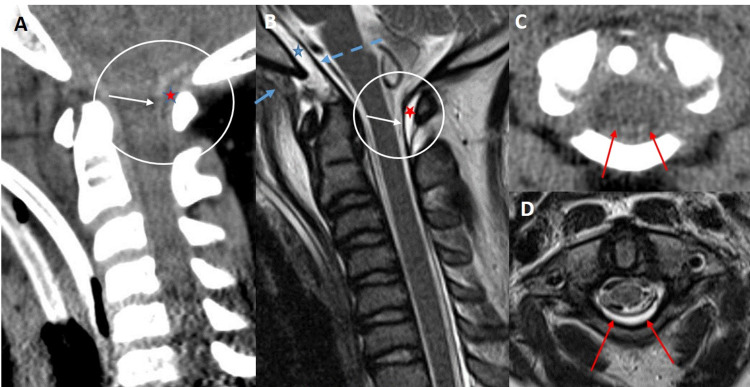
9-year-old female status post motor vehicle accident presenting for cervical CT and MRI exam. Sagittal CT (A) and T2 weighted MRI (B) of the craniocervical junction demonstrate a stripping injury of the posterior atlanto-occipital membrane from the posterior C1 arch (white arrow) with a localized posterior epidural hematoma at C1-C2 (red star). Associated stripping injury of the tectorial membrane (blue dashed arrow) from the posterior clivus with a retroclival epidural hematoma (blue star) with posterior subluxation of C1-C2 in relation to the skull base.  Partial tear of the anterior atlanto-occipital membrane (short blue arrow) is also present. Axial CT (C) and T2 weighted MRI (D) images demonstrate the posterior epidural hematoma localized between the posterior dura and posterior C1 arch (red arrows). CT: computed tomography, MRI: magnetic resonance imaging
